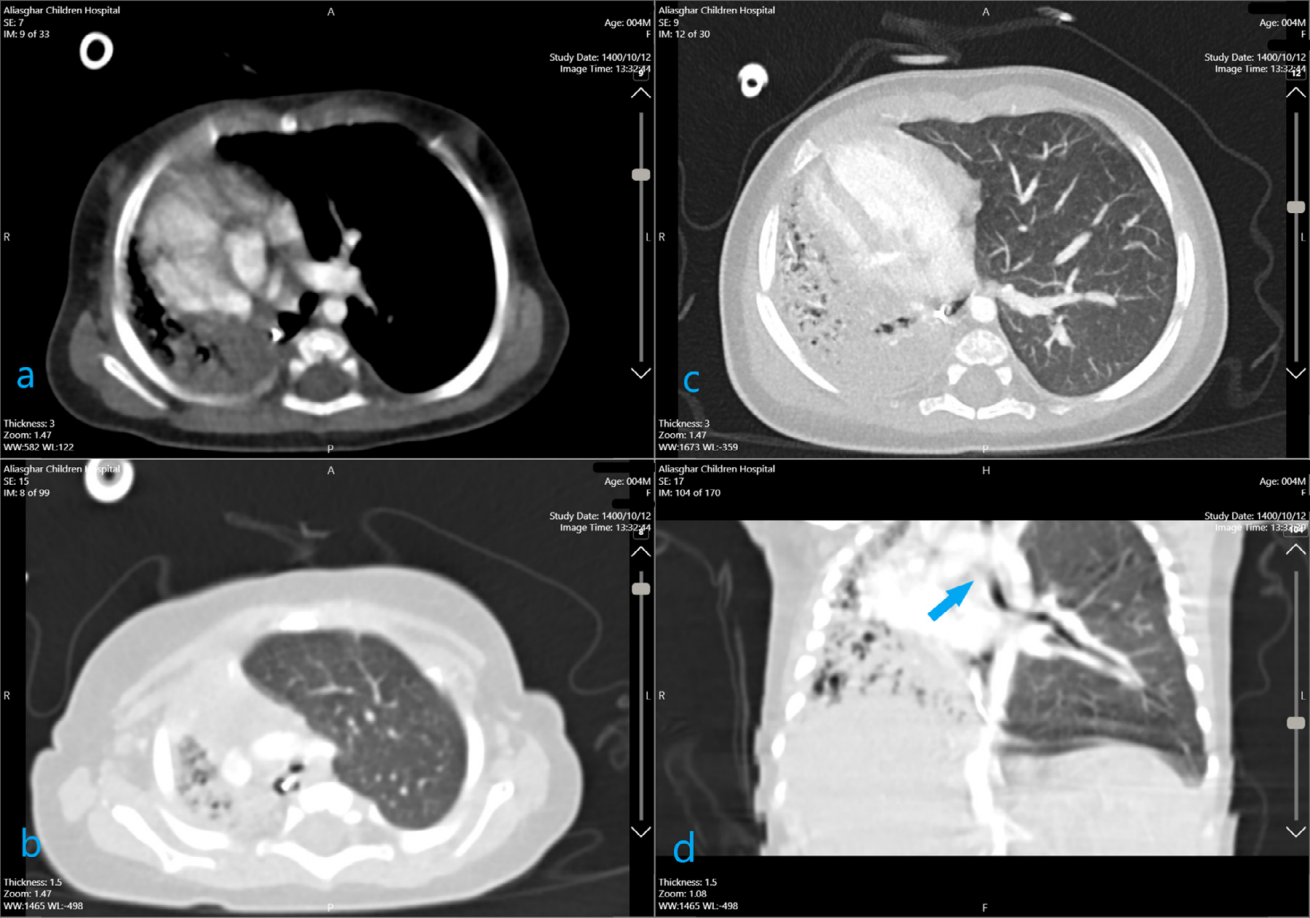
IV contrast chest CT scan shows a hypoplastic right lung with hypoplastic normal origin pulmonary vessels (a). No clear connection is seen between the esophagus and the right main bronchus (b, c) but the right bronchus was absent at the level of the carina (arrow in d) and is seen almost at the base of the right hemi thorax on the right side of the esophagus.
